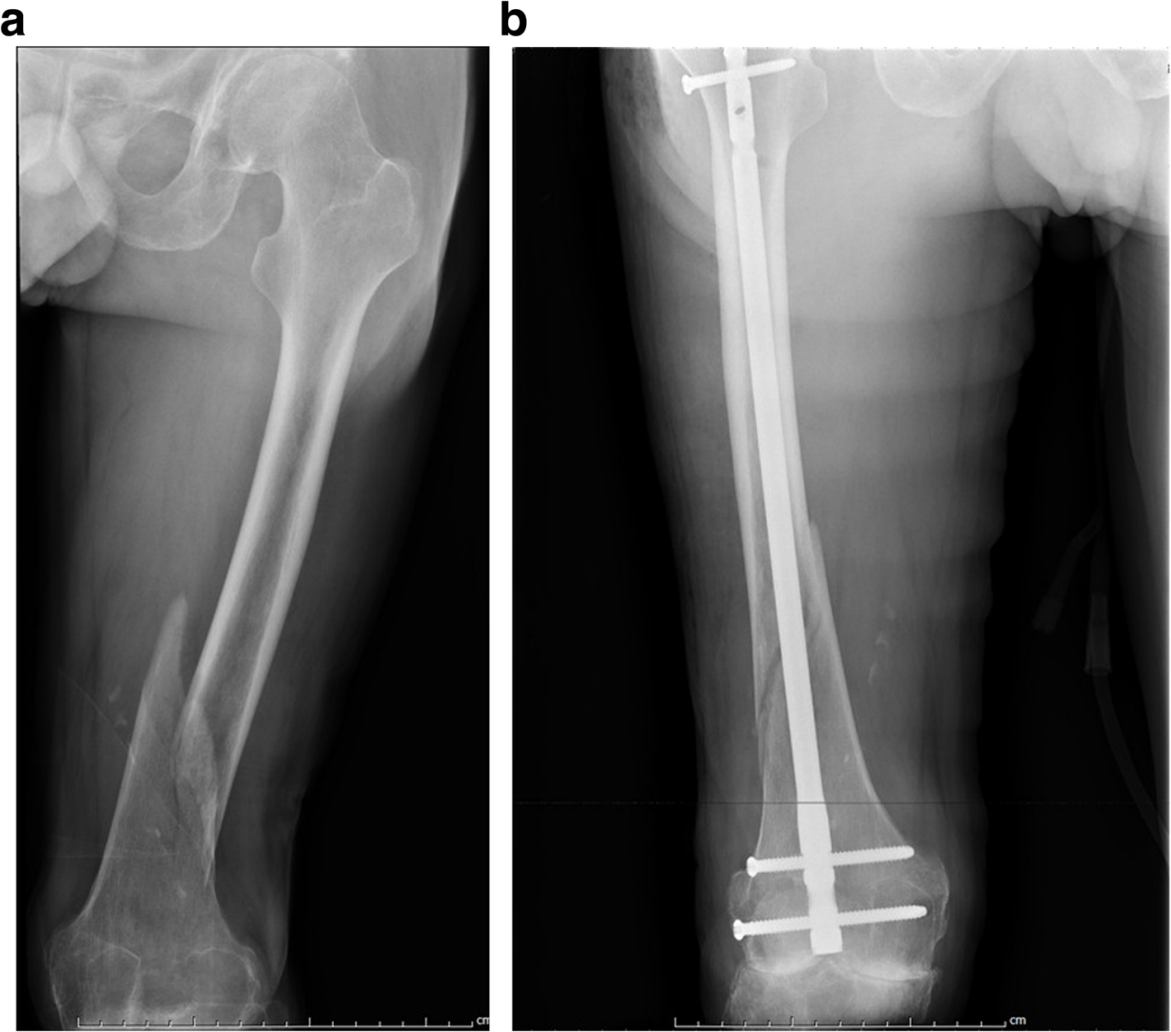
A 45-year-old patient sustained a right distal femur fracture. a Preoperative X-ray. b Postoperative X-ray
